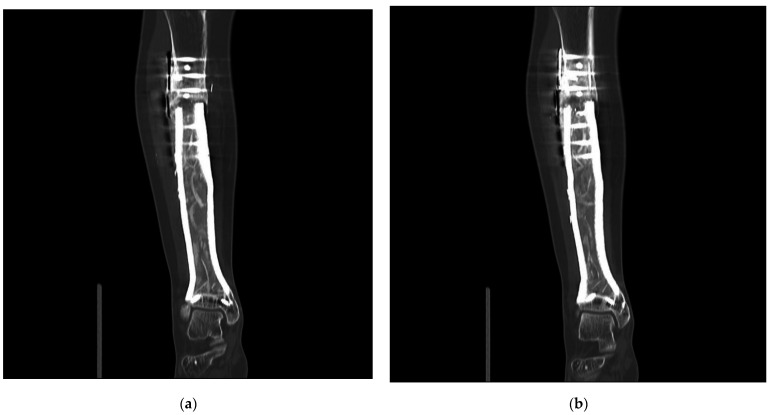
Patient #1: Computed tomography scan 43 months after primary reconstruction: (a,b) coronar view of the implant with the depiction of the bone graft-loaded hollow implant cavity and persistent non-union of the proximal implant-bone interface.
